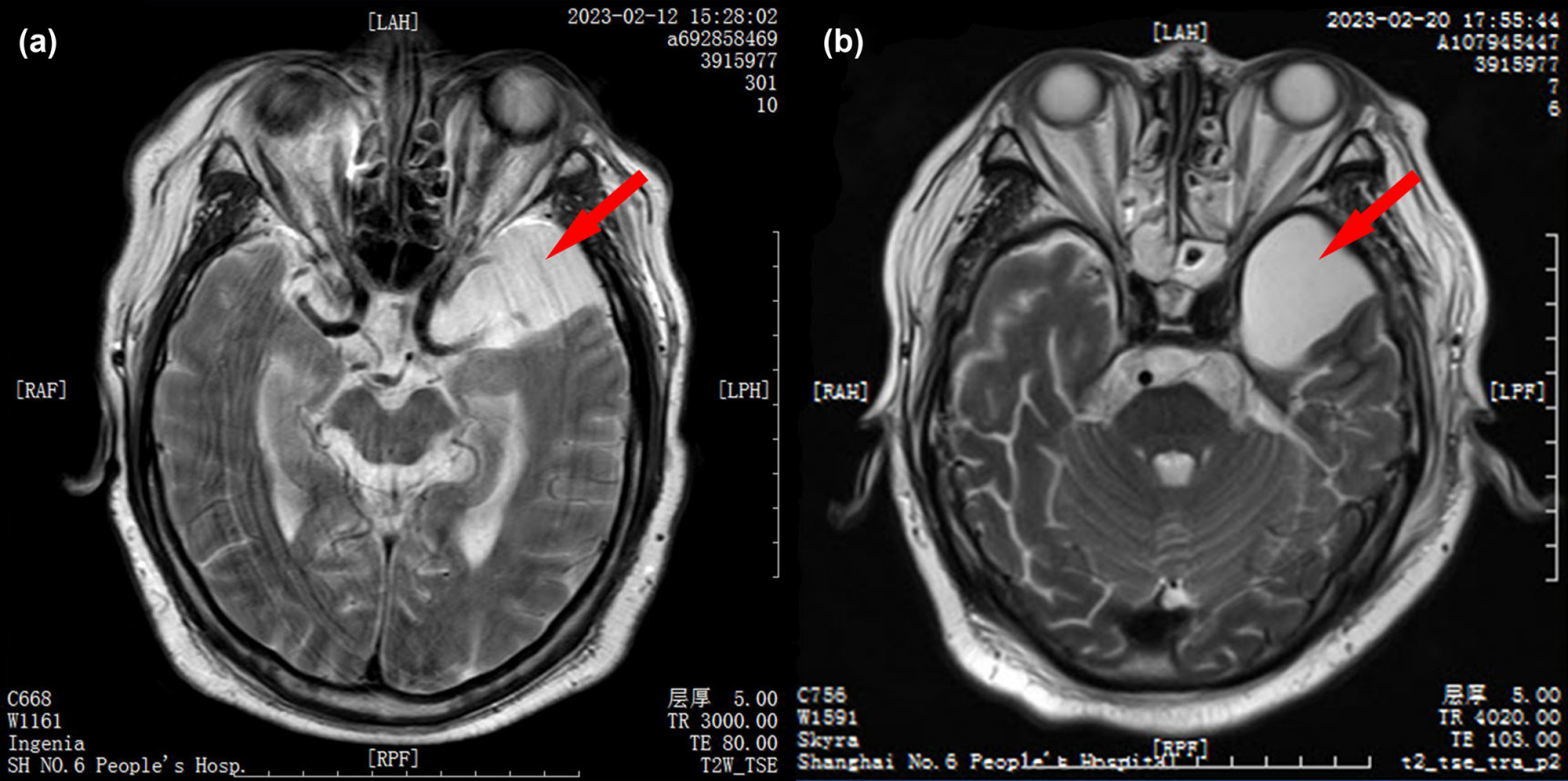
MRI demonstrated: (a) the third day after admission (12 February) and (b) Day 11 after admission (20 February). The results showed that *LM* infection did not cause parenchymal changes in the patient. The red arrow refers to the patient’s old cyst lesion.
